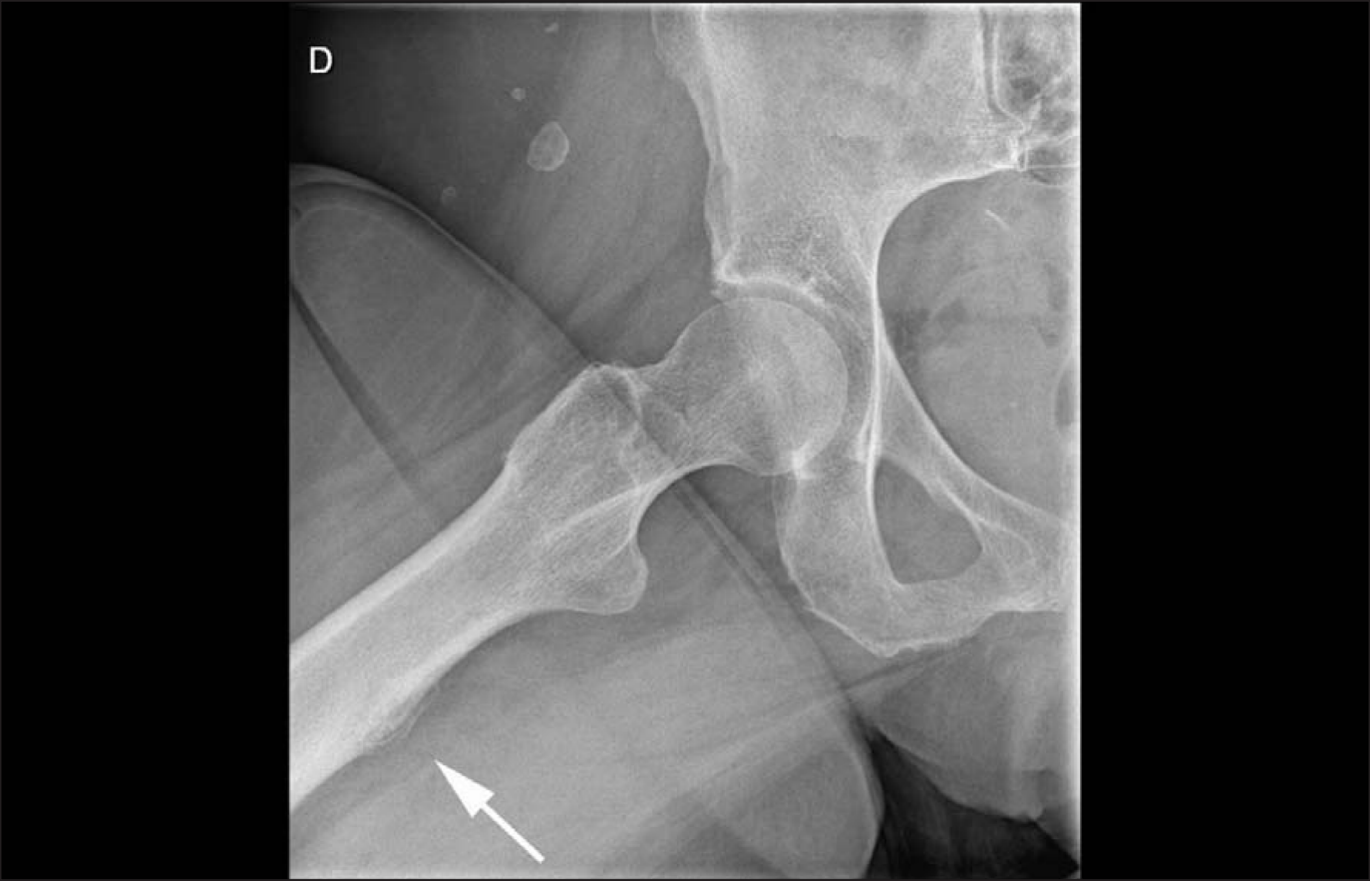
Plain radiograph of the right hip showing a mature periosteal reaction along the posteromedial proximal femoral diaphysis (arrow), without any fracture identified.
